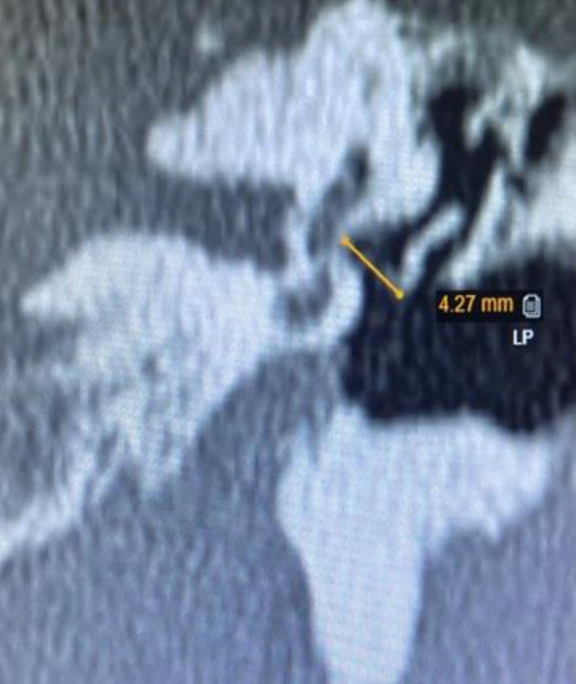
HRCT Imaging measuring a long process of incus to oval window
